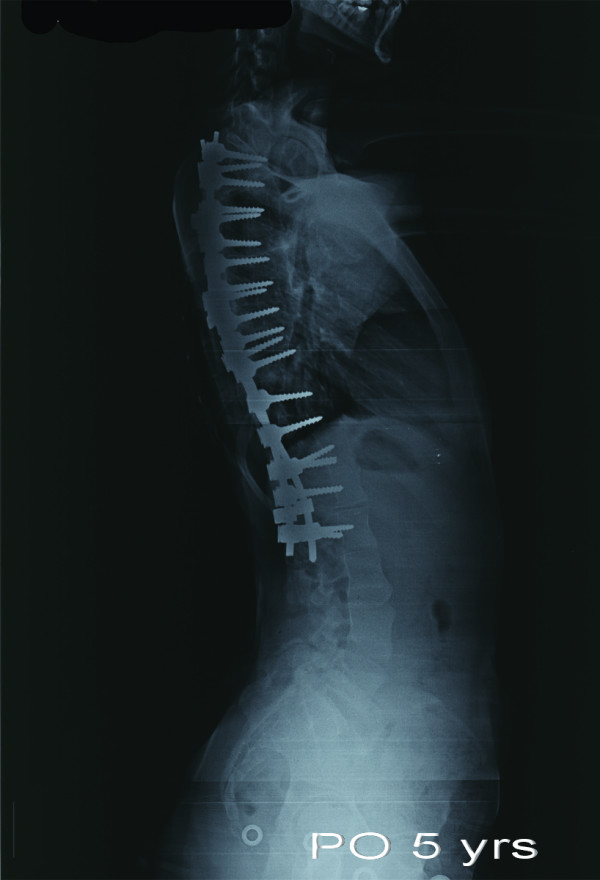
Postoperative lateral radiograph taken 5 years after surgery. Thoracic kyphosis angle was reduced to 10.1°.
